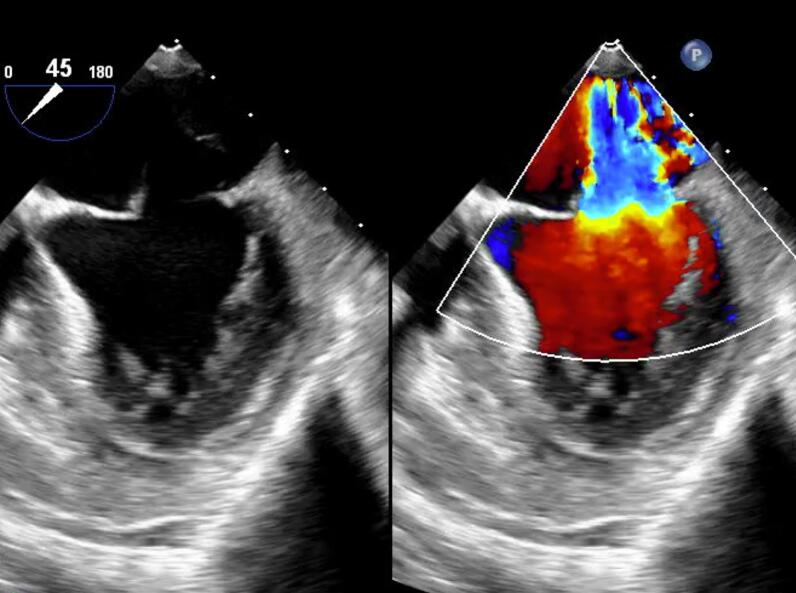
Transoesophageal echocardiography demonstrates mitral regurgitation caused by rupture of the anterior papillary muscle of the left ventricle.
